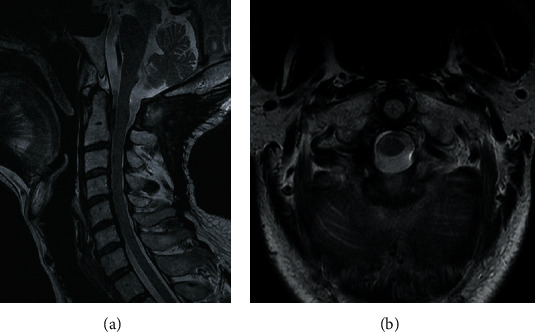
(a, b) T2-weighted sagittal and axial MRI at one year after surgery showed no obvious recurrence.
